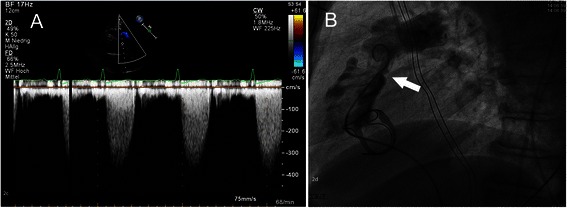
Transthoracic echocardiography and angiography of patient no. 2. A: Transthoracic echocardiography of the degenerated pulmonal valve prosthesis in continuous wave doppler with signs of stenosis. B: Angiography of the bioprosthesis with stenosis of the proximal anastomosis (see marker).
